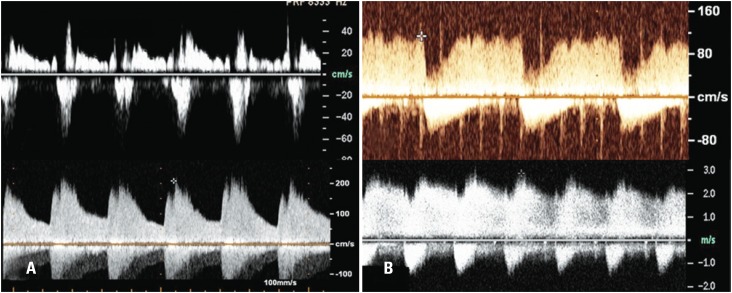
Transductal flow pattern on pulsed Doppler^(13)^. (A) Hypertension: bidirectional flow from the pulmonary to the aorta, on early systole (below baseline), followed by a small flow from the aorta to the pulmonary (above baseline). (B) Growing: bidirectional flow, however with decreased flow from the pulmonary to the aorta and increased flow from the aorta to the pulmonary (C) Pulsatile: flow only from the aorta to the pulmonary, pulsatile, with peak velocity of approximately 1.5m/s. (D) Closing: flow directed from the aorta to the pulmonary, not pulsatile but rather continuous, with a higher peak velocity
